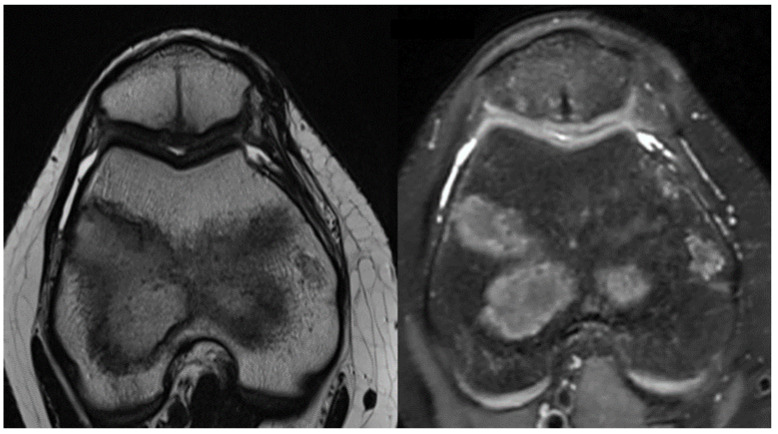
Six months follow-up MRI images that show complete filling of the osteochondral lesion of the patella.
